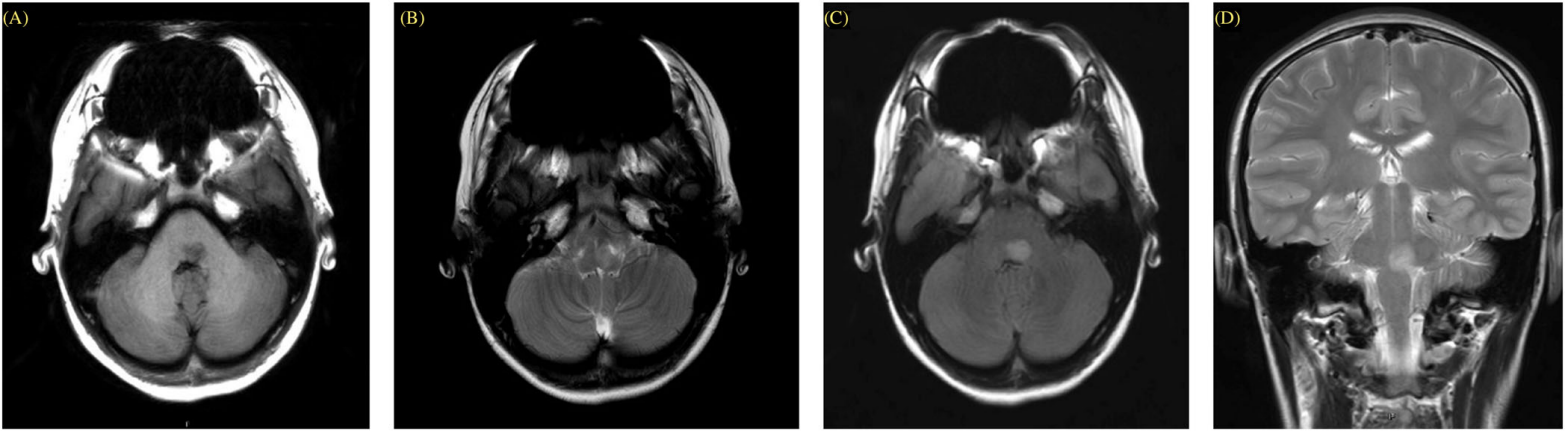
Initial magnetic resonance imaging (MRI) of brain stem leukoencephalopathy (Case No. 5). (A) Axial longitudinal relaxation time‐weighted imaging (T1W1), (B) axial transverse relaxation time‐weighted imaging (T2WI), (C) axial T2/fluid‐attenuated inversion recovery (FLAIR), and (D) coronal T2WI. Well‐defined border lesion was shown at left pontomedullary junction, which is hypointense on T1WI and hyperintense on T2WI/FLAIR. This lesion causes mass effect to the fourth ventricle.
